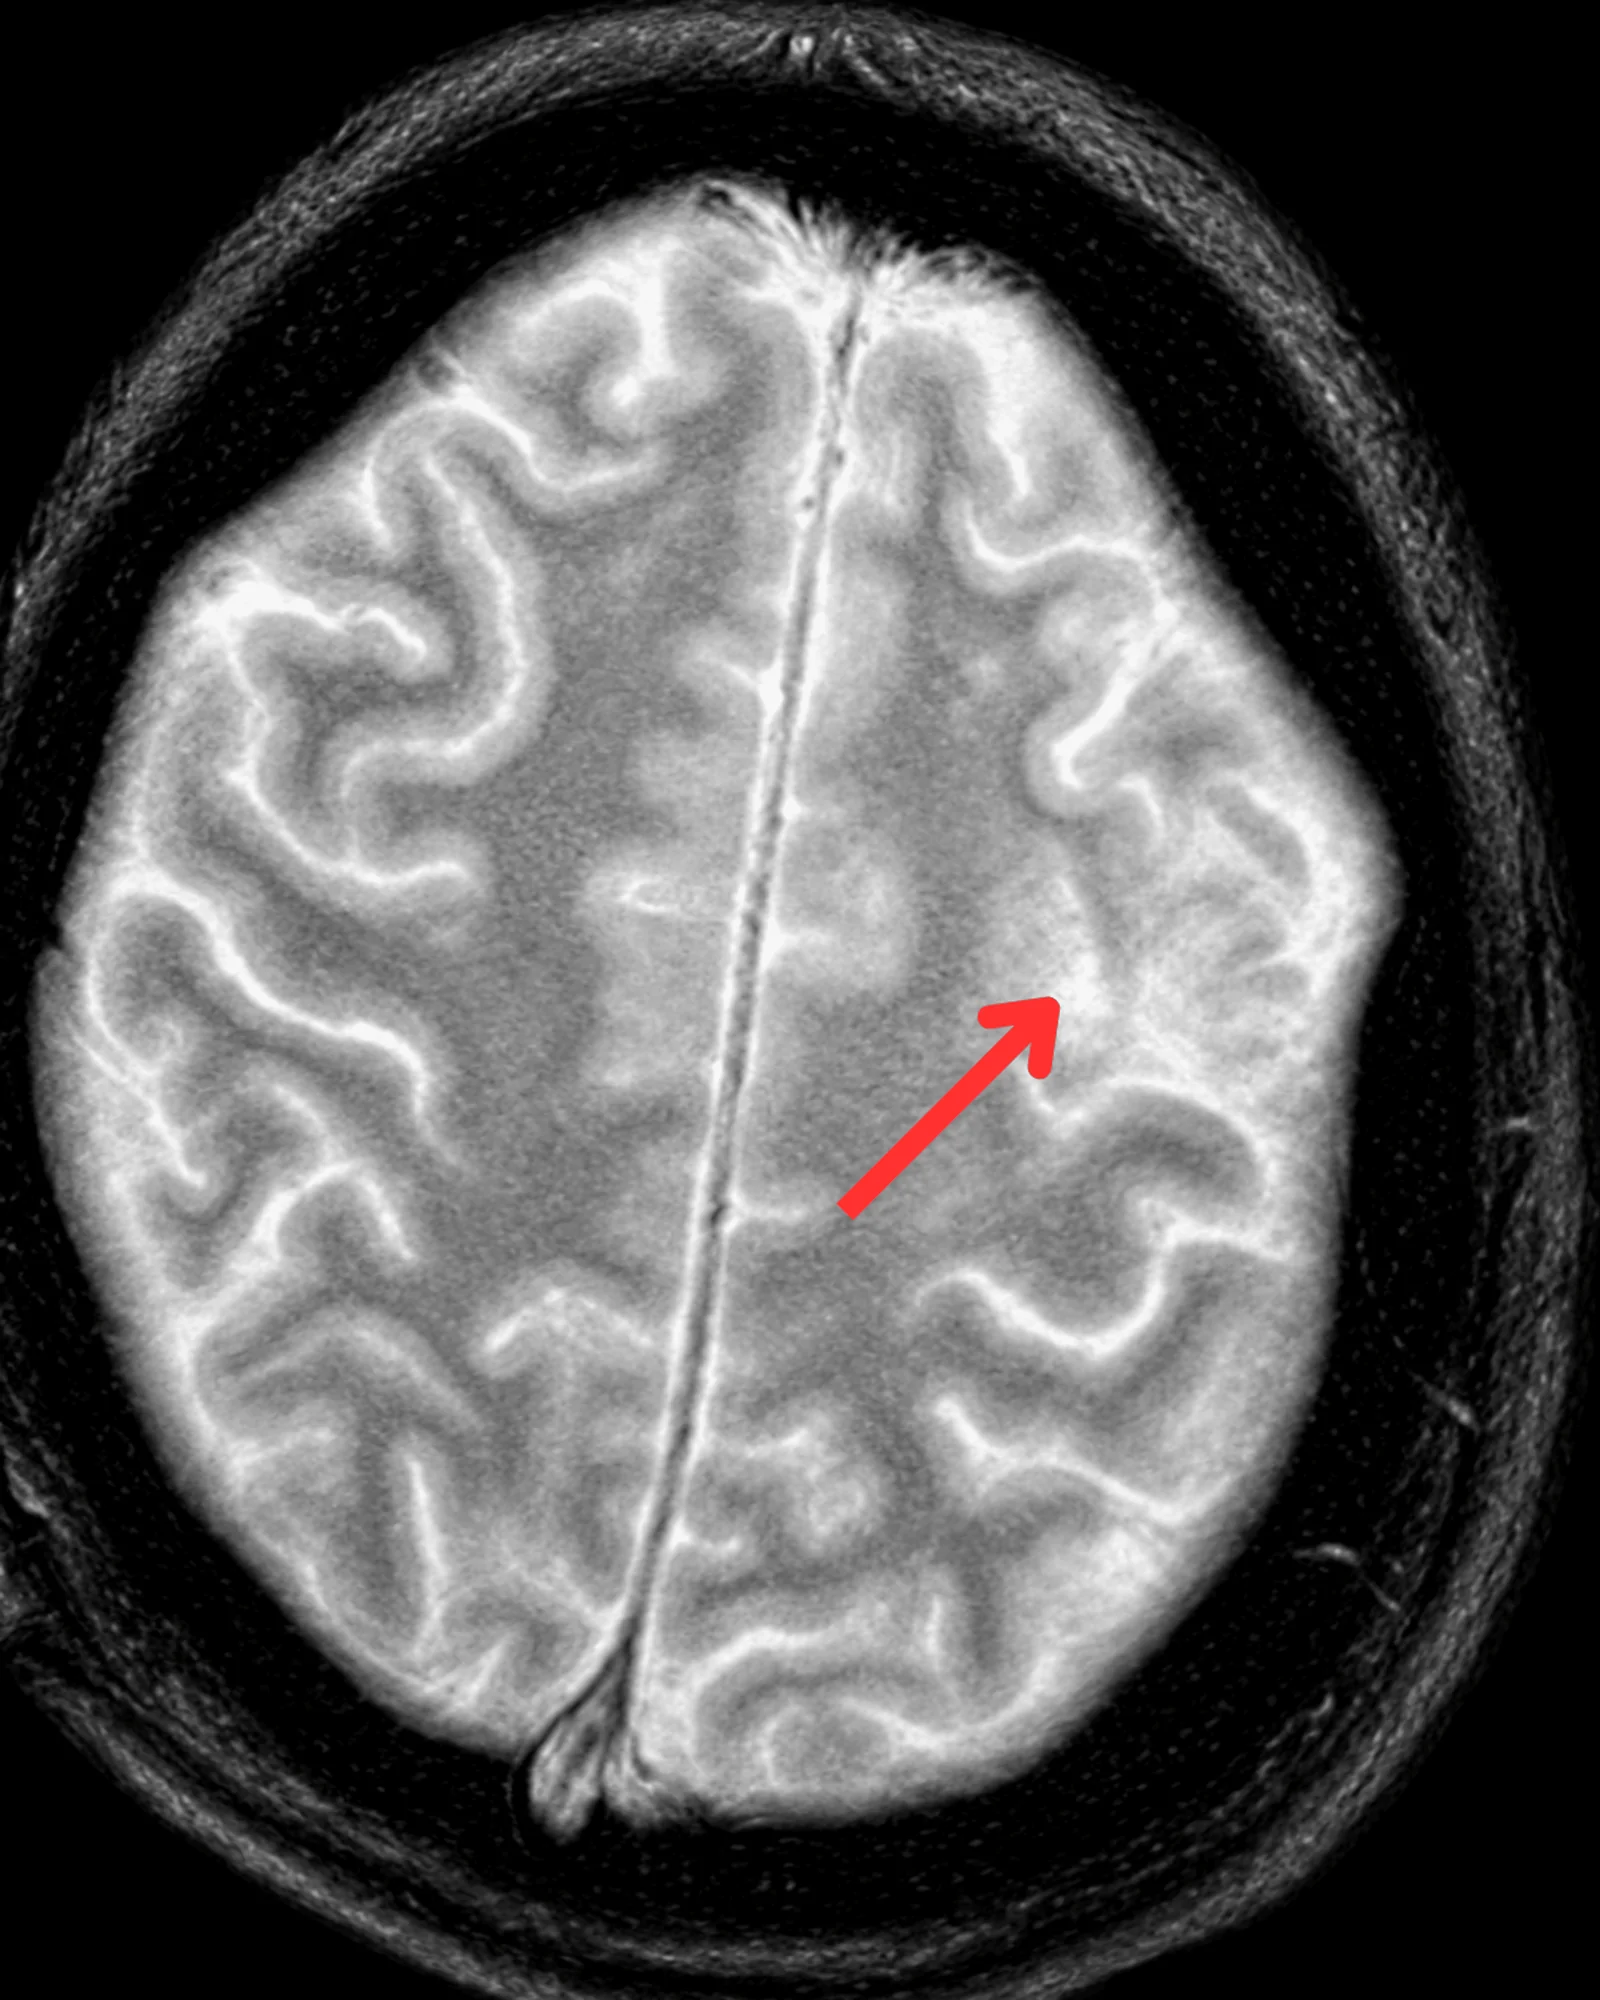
Brain MRI on admission showing no acute intracranial pathology, with findings limited to chronic changes consistent with prior infarction. Imaging may be normal in early West Nile encephalitis.
